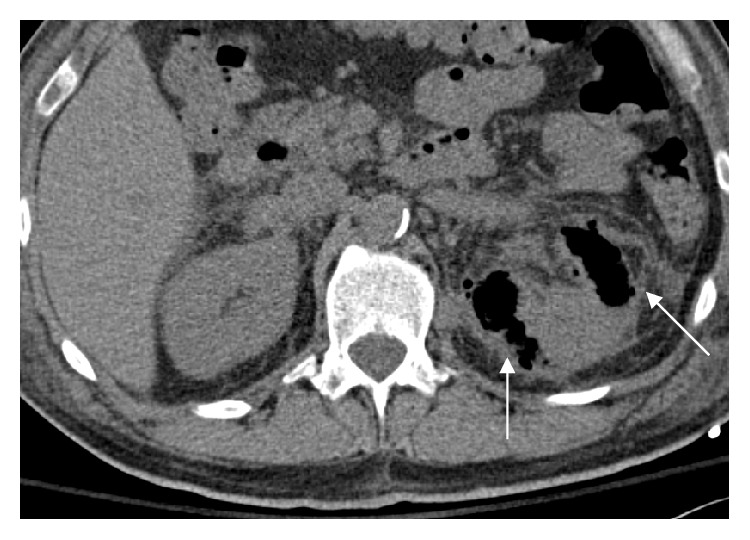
Axial noncontrast CT scan of the abdomen showing air (arrows) in the left renal parenchyma.
